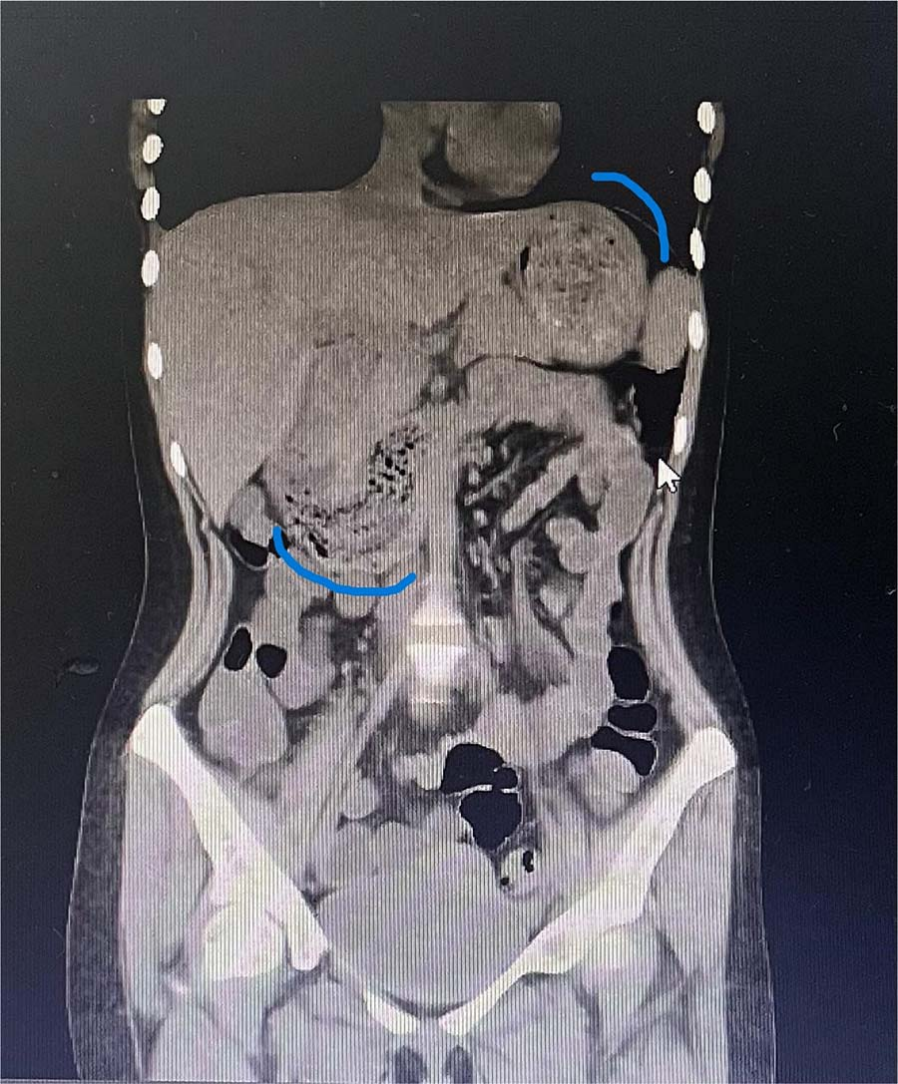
Sagittal CT image showing the foreign body occupying the gastric cavity and extending into the third and fourth portions of the duodenum.
